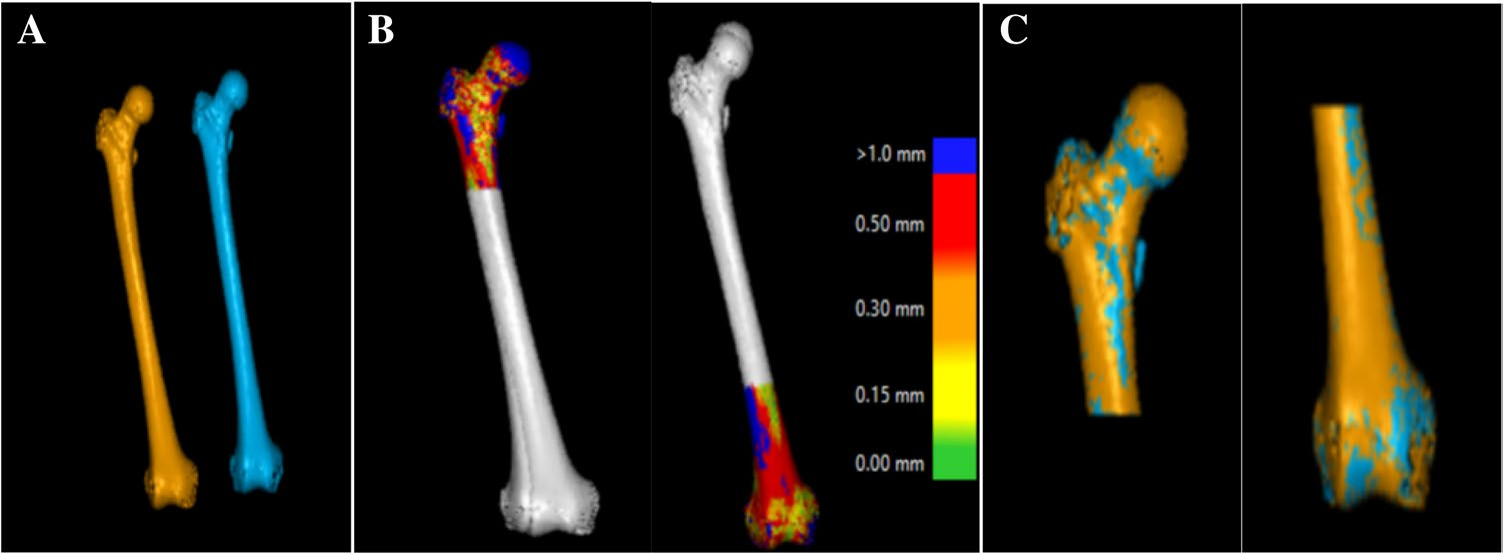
Stages of volume fusion of the proximal and distal femur from (a) the original right femur and the mirrored left femur. b The color scale indicates how closely the software could place the object of interest in the two CT stacks for the proximal part and the distal part, respectively. c The proximal femur is assumed as the static portion (reference) to report rotation and translation of the distal femur
